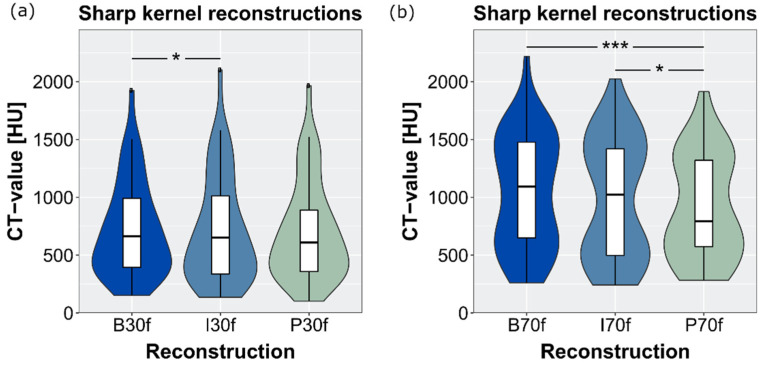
CT values measured from stones in (a) soft kernel reconstructions (filtered back-projection (B30f), iterative reconstruction (I30f) and PixelShine (P30f)) and (b) sharp kernel reconstructions ((filtered back-projection (B70f), iterative reconstruction (I70f) and PixelShine (P70f)). * *p* < 0.050, * *p* < 0.001.
